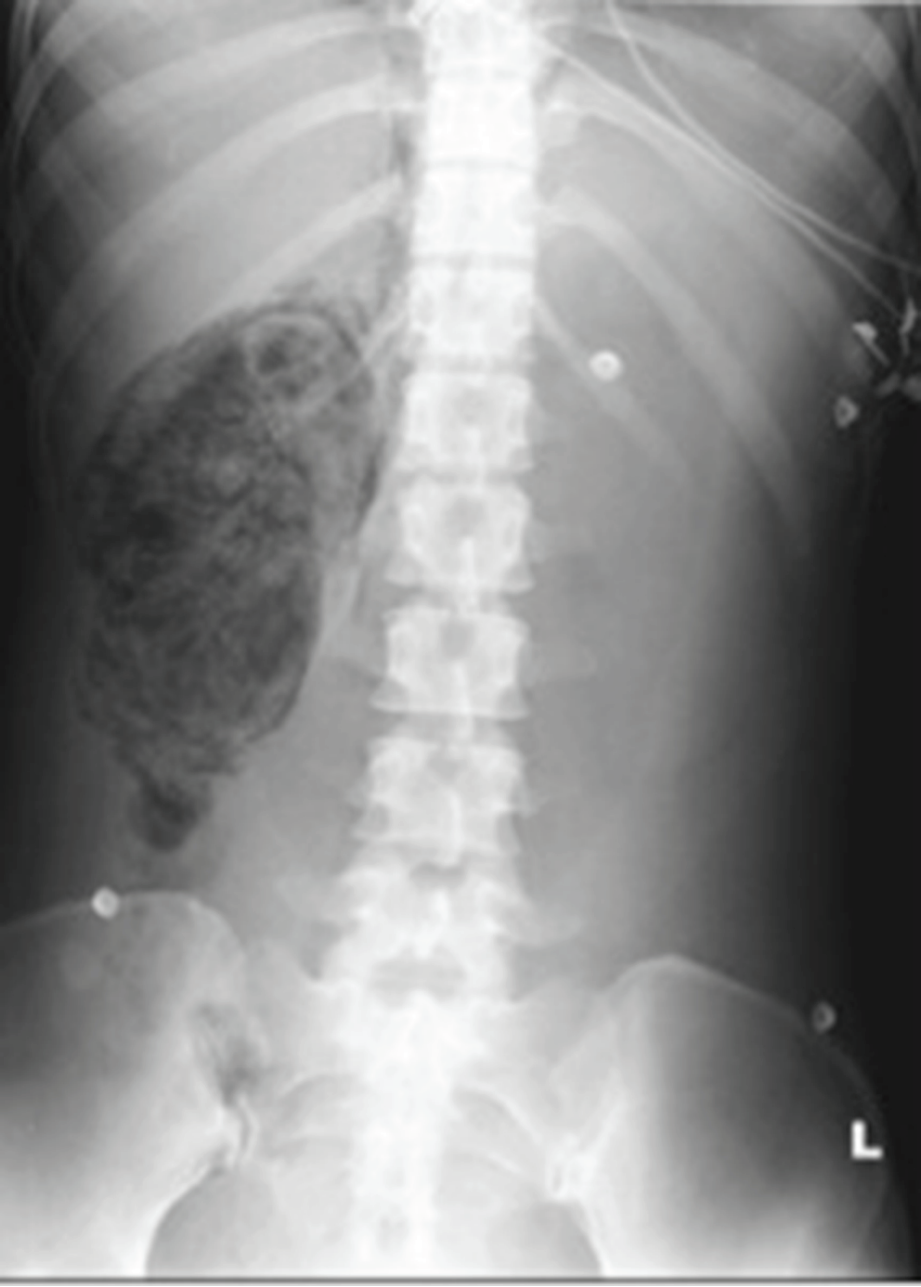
Chest X-ray demonstrating subdiaphragmatic free gas.
